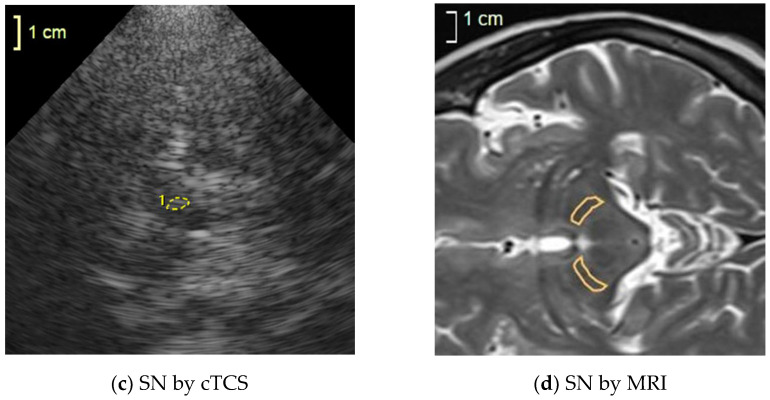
Regions of interest (ROI) examples in axial scanning plane images co-registered by different methods for the same healthy participant: (a) half of the midbrain in radiofrequency (RF) transcranial sonography (TCS) data-based B-mode image, (b) analyzed half of the midbrain and not-analyzed half of the midbrain mapped as root mean square (RMS) of confident displacements detected from RF TCS signal sequences; (c) substantia nigra (SN) in conventional TCS (cTCS) image of midbrain (d) SN by magnetic resonance imaging (MRI) of midbrain, T2W/TSE/2 mm sequences. Contours of ROI depicted in colorful lines (see online version). In sonography examples, the ultrasound transducer was on the left side of the head or at the top of images.
